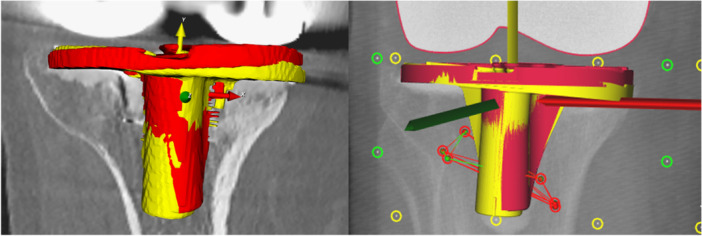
Visualization of the patient with the largest MTPM between 1 year (red) and 5 years (yellow) postoperative measured with CT‐RSA: 5.74 mm (Left), and visualization of the same patient with the largest MTPM between 1 year and 5 years postoperative measured with RSA: 5.07 mm (Right), The migration coordinate system is shown, with *X*‐axis (red, positive = medial translation), *Y*‐axis (yellow, positive = proximal translation) and *Z*‐axis (green, positive = anterior translation); MTPM = maximum total point motion; mm = millimeter. Note that the exact location and the orientation of the CT‐RSA and RSA coordinate systems vary slightly.
